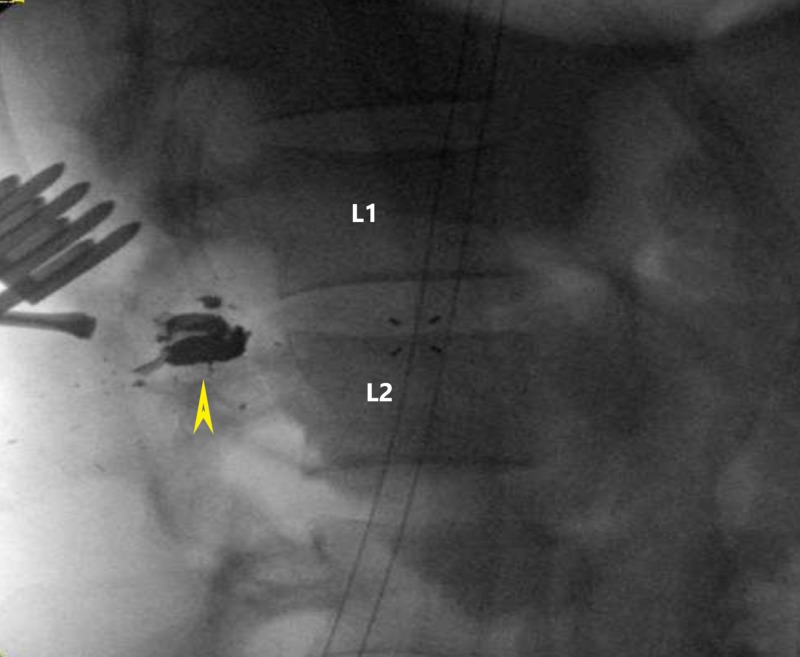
Intraoperative X-ray revealing the location of the bullet fragments (yellow arrowhead) and their anatomical relation to L1-L2, canal, and neuroforamen.
